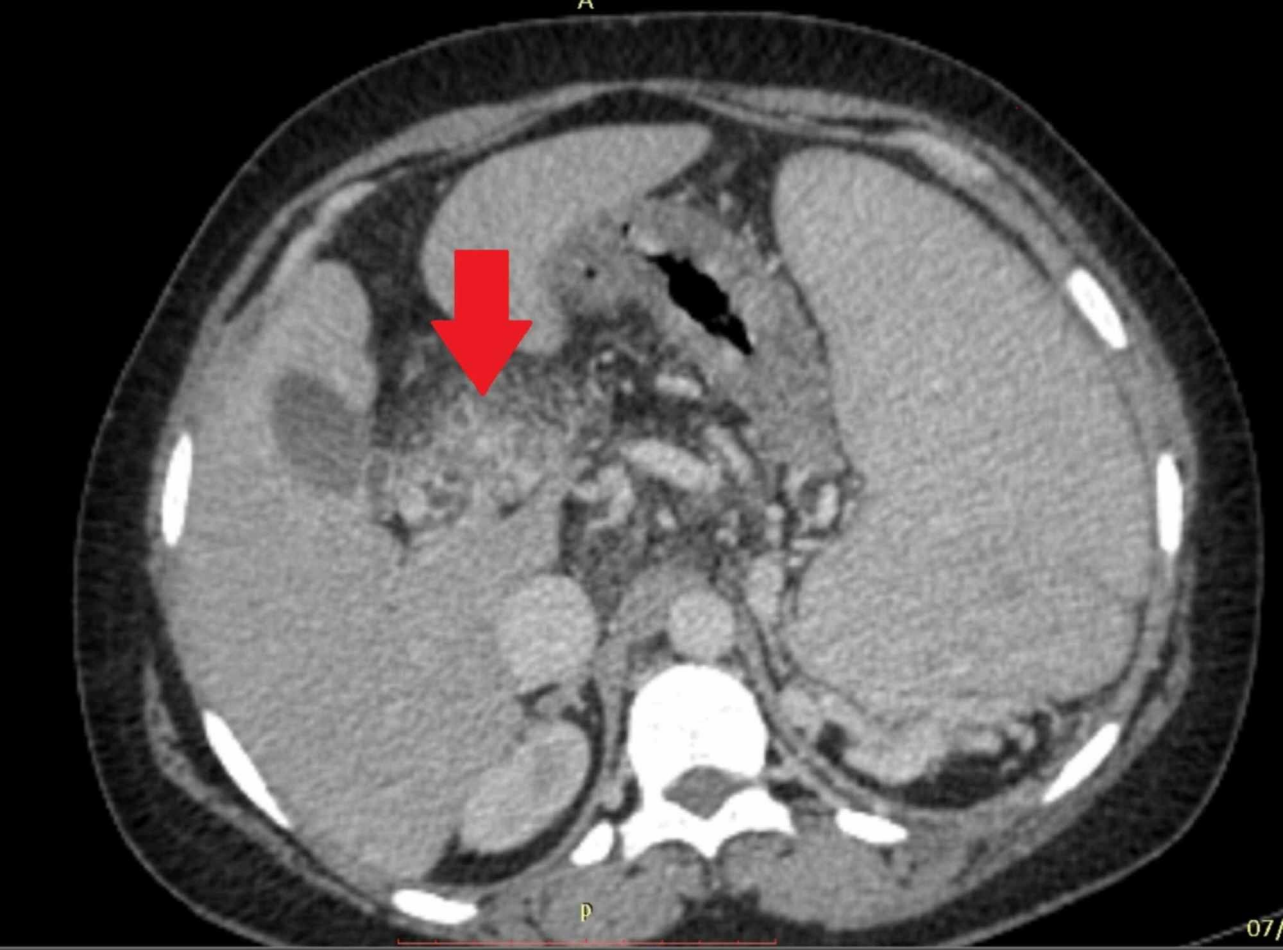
CT scan of abdomen (axial view) showing collaterals or cavernoma formation around PV CT, computed tomography; PV, portal vein
